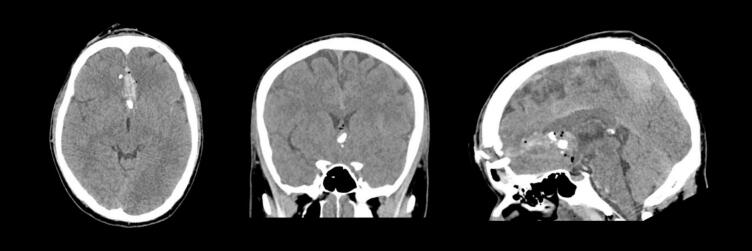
Preoperative non contrast CT Brain (Axial/Coronal/Sagittal views).
